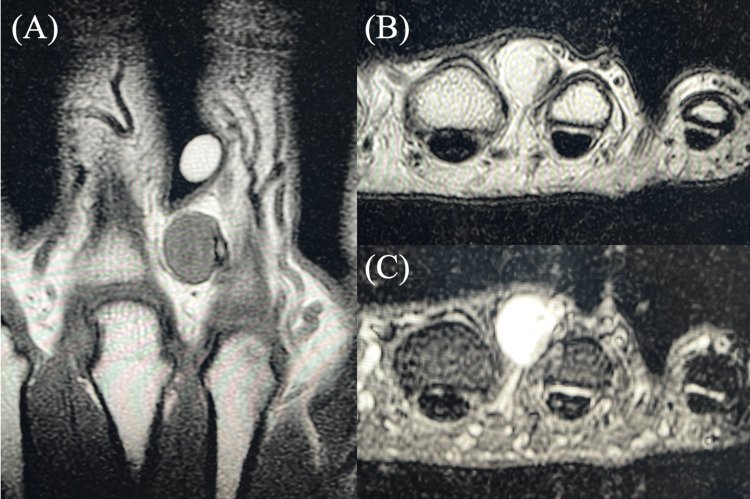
Preoperative MRI findings for case 2. A tumor with well-defined borders is present between the index and middle fingers. (A) Isointense signal on a coronal T1-weighted image. (B) High signal intensity on an axial T2-weighted image. (C) High signal intensity on an axial STIR image. STIR: Short tau inversion recovery
